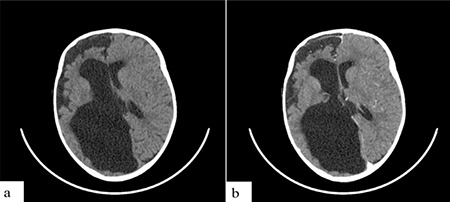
Axial non-contrast-enhanced (a) and contrast-enhanced (b) computed tomography of the brain showing severe atrophy of the right cerebral hemisphere with an expansion of the cerebrospinal fluid space over the ipsilateral cerebral convexity and dilatation of the lateral ventricle on the same side (the so-called “exvacuo hydrocephalus”). No areas of abnormal contrast enhancement
